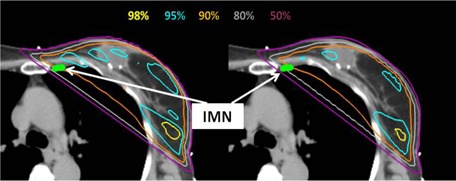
An example of the planned (left) and delivered (right) axial CT images demonstrating changes in the IMN coverage. The prescription was 200 cGy×23. The mean RTDs are (0.06, 0.15, 0.78, 0.68, −0.57, 2.76), and the SDs of the R TDs are (1.01, 1.41, 0.78, 0.52, 0.25, 0.44).
